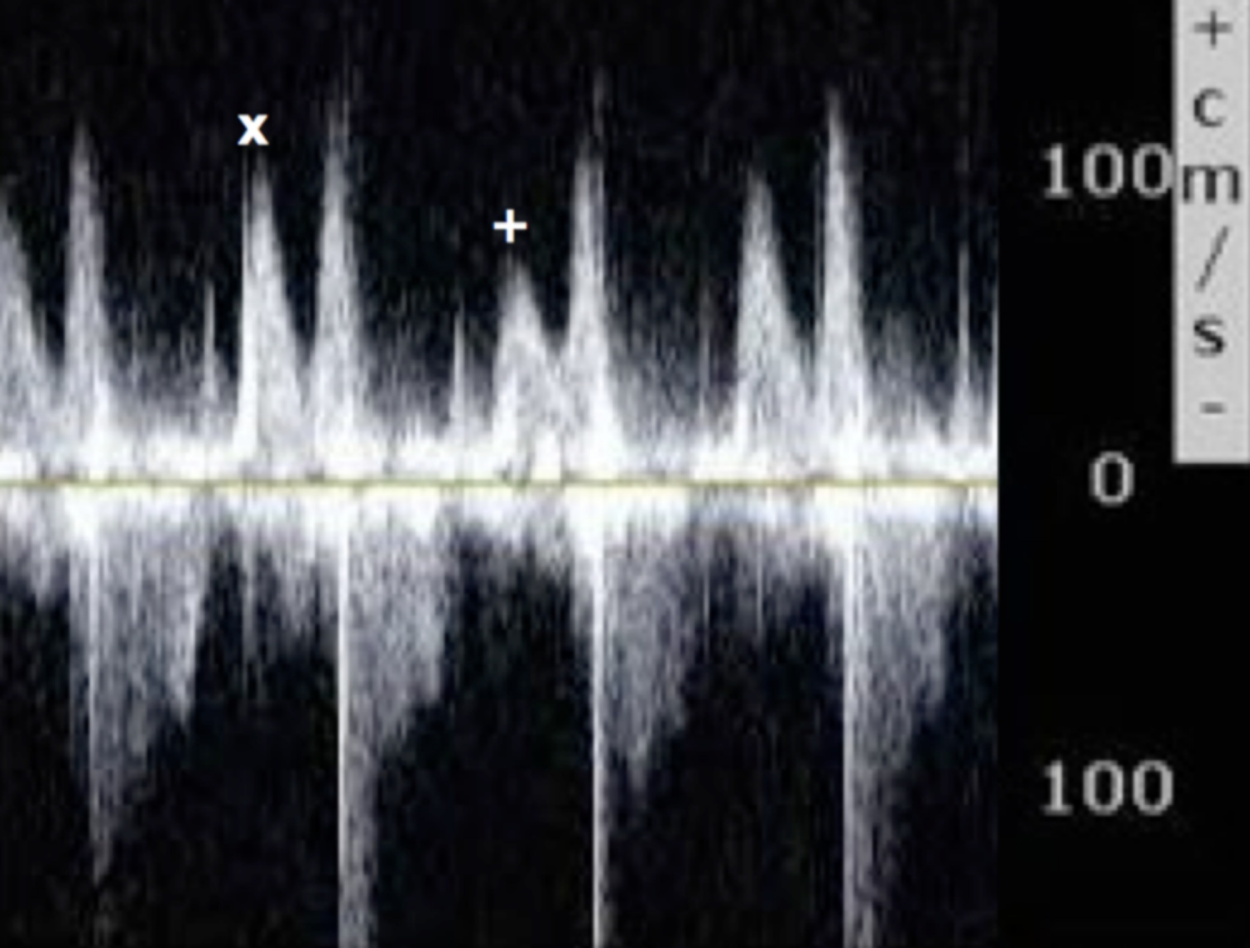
Echocardiogram; pulse wave Doppler across the mitral valve over consecutive beats. Revealing respiratory variation in mitral inflow emptying velocity between expiration (x) and inspiration (+) >25% suggestive of ventricular interdependence.
